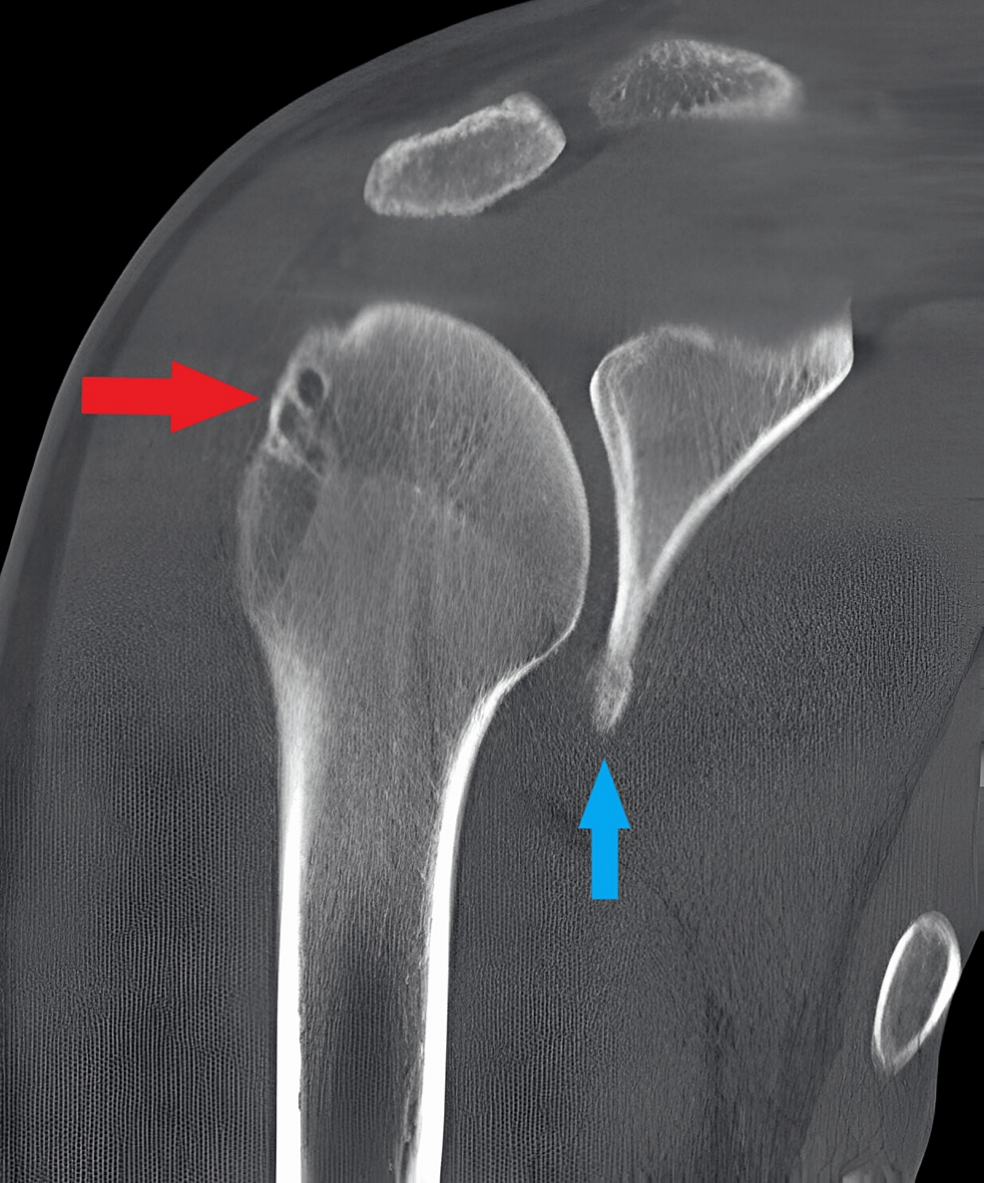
CT scan coronal view of right shoulder showing Bankart lesion (blue arrow) and Hill-Sach lesion (red arrow).
